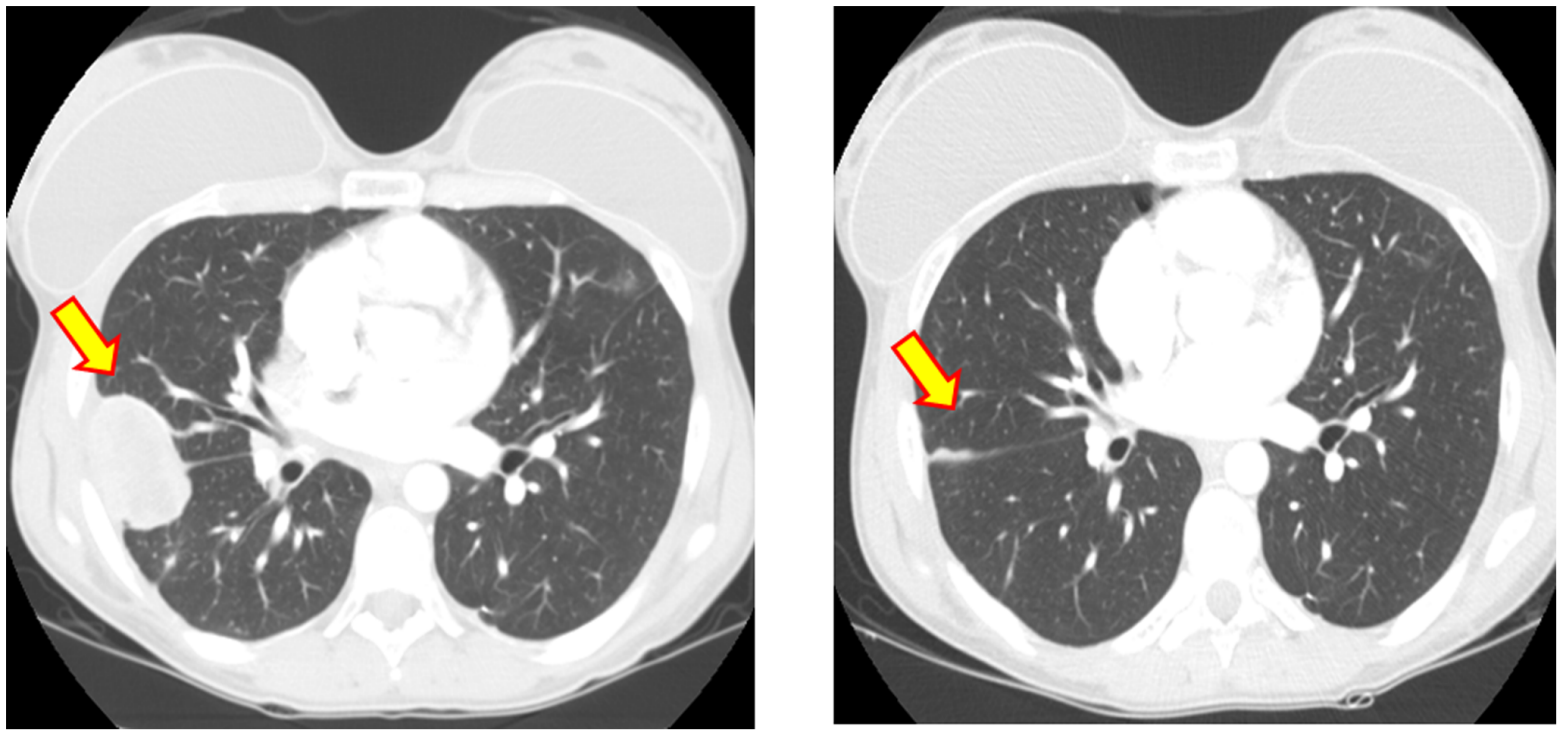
CT of the chest in Case # 2 with Ewing's sarcoma response to single agent IGF1R antibody alone [11]. Left panel shows lung metastases before IGF1R antibody therapy and right panel shows response to therapy after six weeks of treatment [11].
